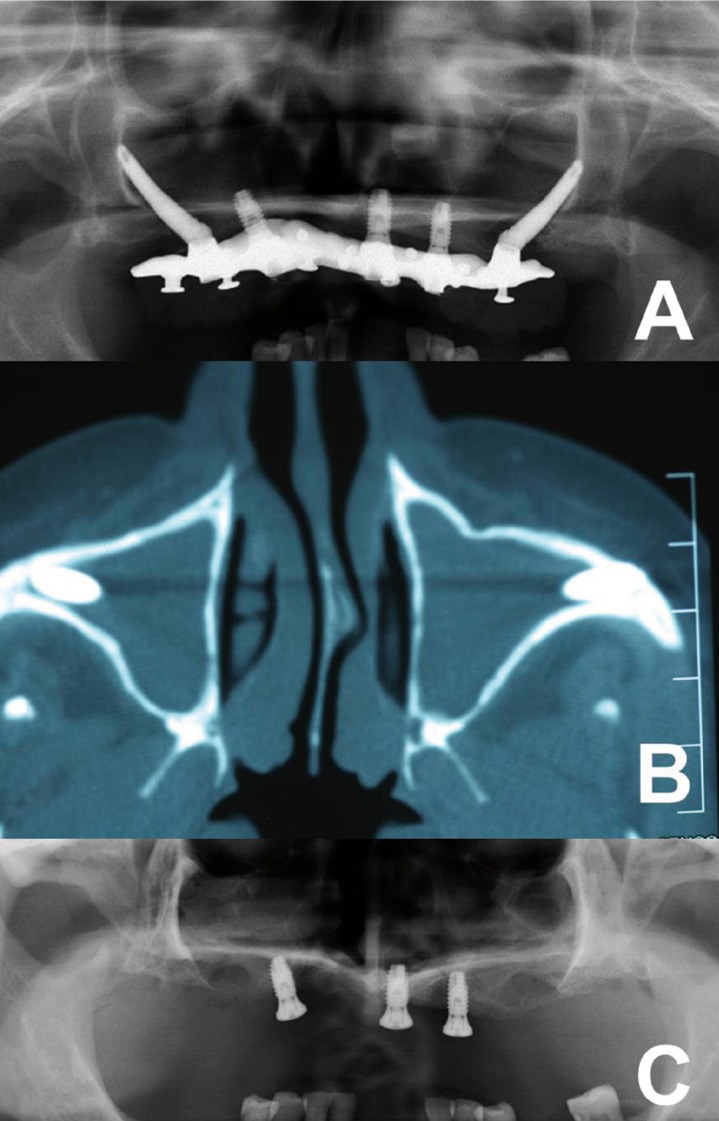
A)Panoramic radiography and B) computerized tomography (TC) showing bilateral maxillary sinus occupation (sinusitis) secondary to permanent oroantral communication due to resorption of the thin palatal bone corresponding to ZIs. C) Control orthopantomography 6 months after surgery.
